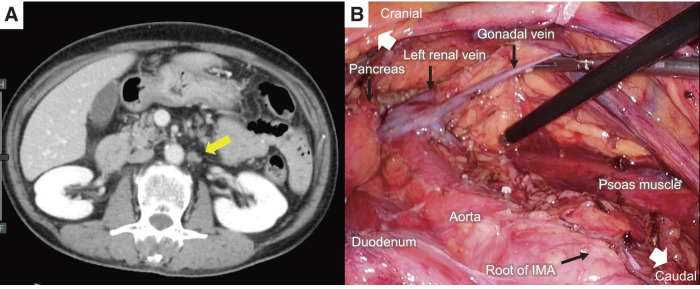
Laparoscopic para-aortic lymphadenectomy for para-aortic lymph node metastasis of rectal cancer. (A) Preoperative enhanced computed tomography image showing para-aortic lymph node metastasis (yellow arrow). (B) Surgical view after para-aortic lymphadenectomy. IMA, Inferior mesenteric artery
